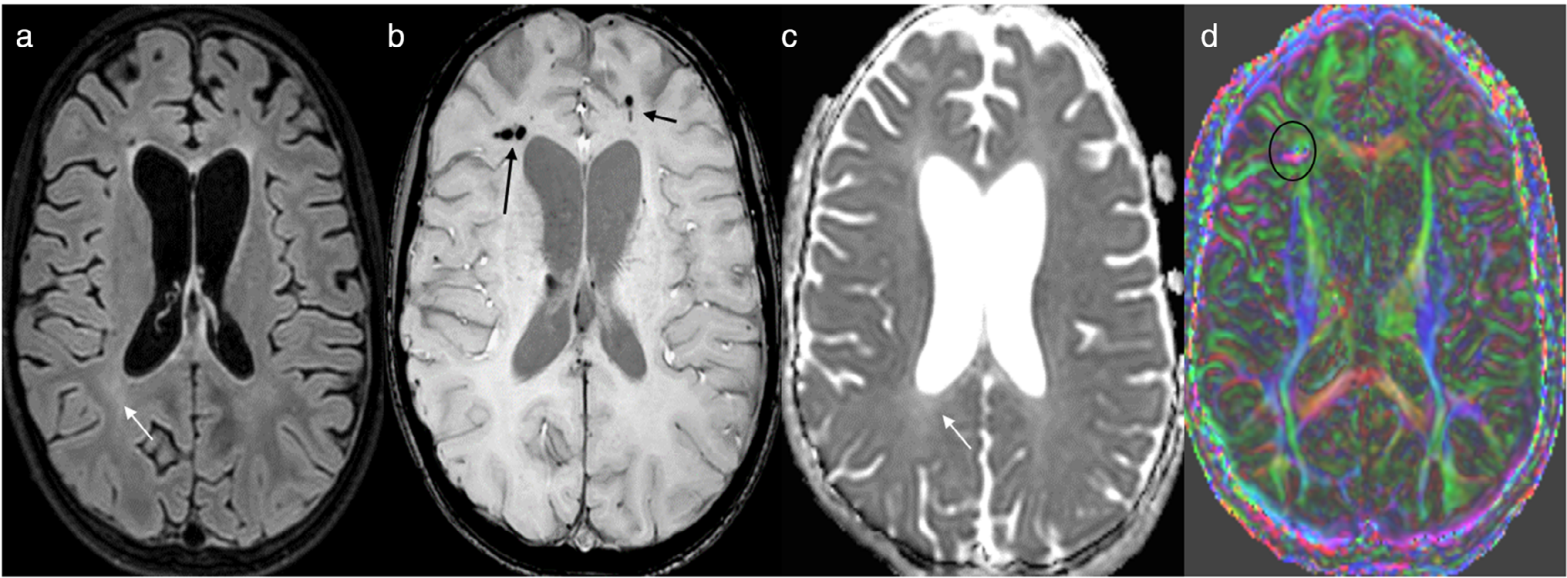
A case study of a 12‐year‐old male with traumatic brain injury: (a) An axial FLAIR image reveals subtle diffuse hyperintensity (white arrow) in the white matter and enlarged ventricles indicative of progressive atrophy. (b) An axial SWI demonstrates multiple hypointensities along the bilateral frontal white matter tracts, reflecting microbleeds (black arrows). (c) Axial ADC maps exhibit increased signal throughout the white matter, confirming elevated extracellular water content (white arrow). (d) A color‐coded FA DTI map highlights disrupted white matter and a thin corpus callosum genu. Additionally, hemosiderin in the right frontal lobe distorts the DTI signal (indicated by a black circle).
